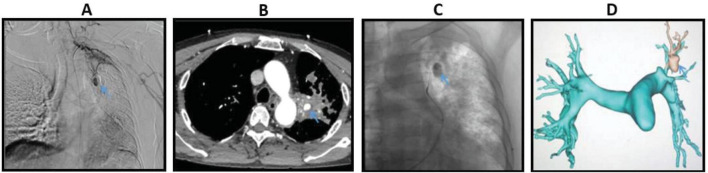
Representative type-C case demonstrating supply-vessel localization under complex dual-supply conditions. (A) Arterial-phase CTA source image(s) of the lesion region used for model inference and reconstruction. (B) Reconstruction-ready 3D-CTA visualization generated from the predicted masks, enabling interactive branch-level tracing of the suspected parent/supply vessel to guide targeted DSA cross-validation. (C,D) Interventional fluoroscopy/DSA view highlighting the angiographic evidence used for reference confirmation.
